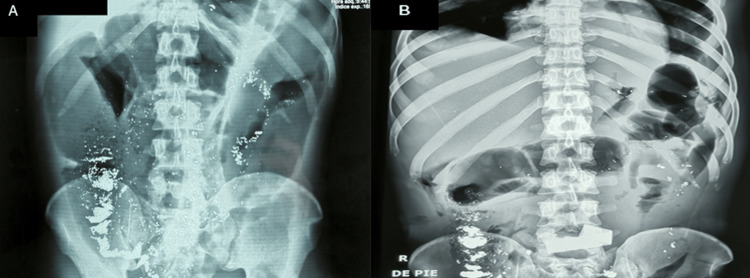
Abdominal X-ray (A) X-ray taken at hospital admission (second day after mercury ingestion). It shows several radiopaque particles throughout the gastrointestinal tract, sequestration in the appendix, and elemental mercury throughout the peritoneal cavity. (B) X-ray taken on the sixth day after mercury ingestion.
